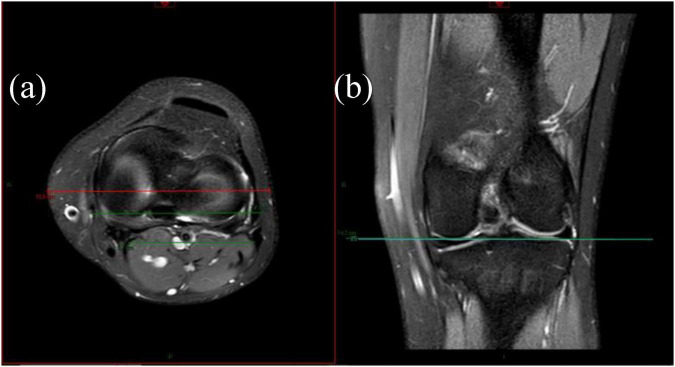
Knee MRI (a) axial and (b) coronal views showing the technique of measuring muscle and fat thickness. Total transverse width was measured from medial to lateral skin margins (red line). The osseous width of the femoral condyles was measured at the same level (green line), and the surrounding soft tissue thickness was estimated accordingly. Muscle thickness was measured at the posterior compartment (short green line).
